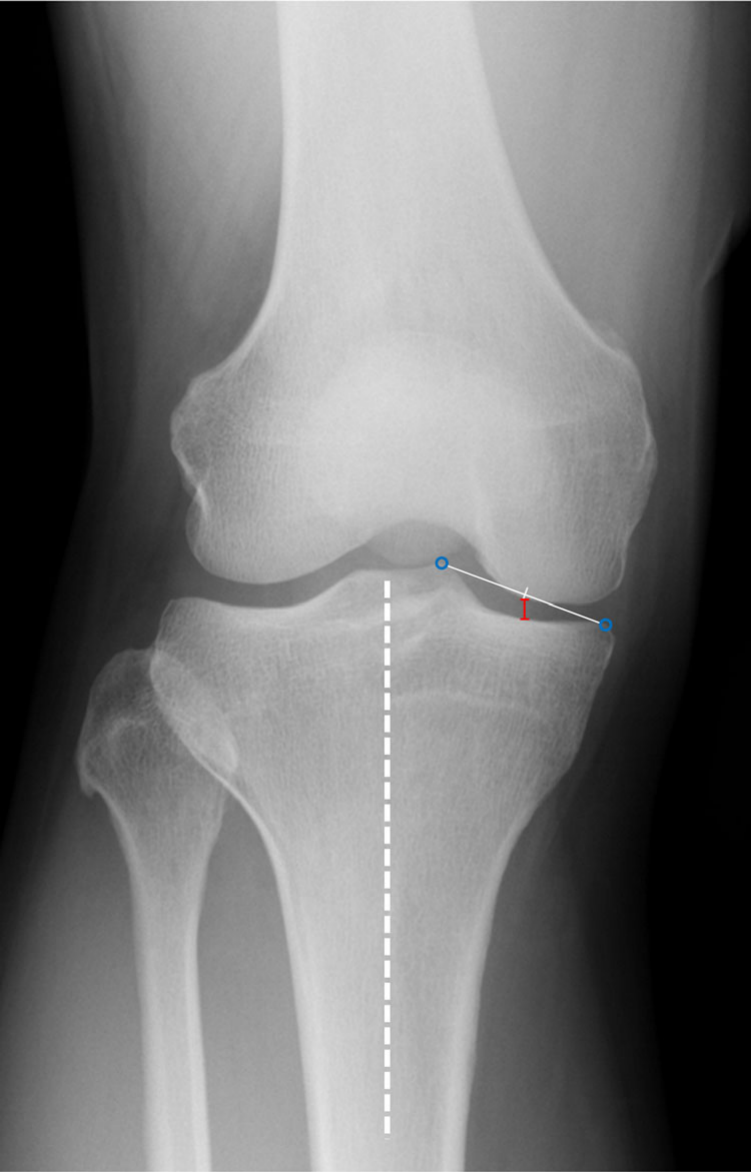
Measurement of the MJS width using FFV radiographs. The MJS width (red line) is measured as the distance between the intersection of the femur and the tibia, a line parallel to the tibial bone axis (white dashed line) at the midpoint between the medial tibial eminence and medial margin of the tibia (blue circles). FFV, fixed‐flexion view; MJS, medial joint space.
